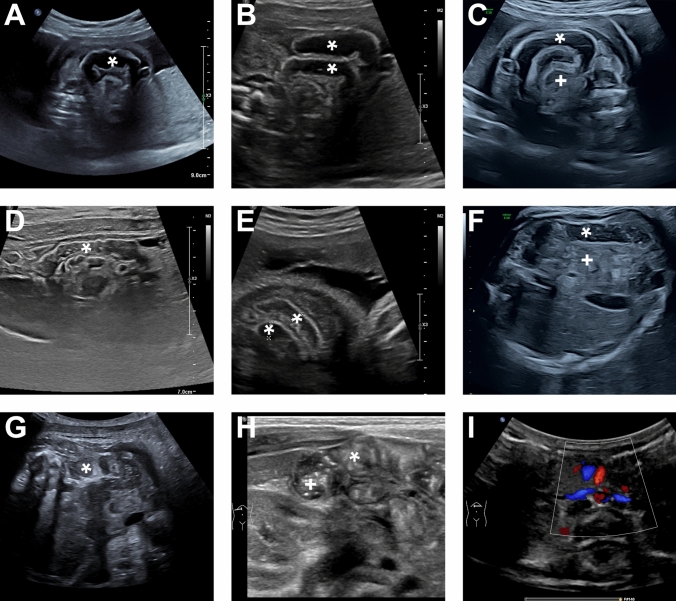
Fetal volvulus with typical prenatal ultrasound findings at 25 weeks of gestation without further symptoms. Due to extreme prematurity, conservative management was the rationale. Sonographic follow-up shows spontaneous detorsion of the volvulus and delivery was at term (38.3 weeks of gestation) without further problems. A Fetal ultrasound at a gestational age of 25 weeks. A dilatation (*) of small bowel loops up to 10 mm was noted in the lower abdomen, with a hyperechogenic bowel wall and detectable peristalsis. The bowel loops are arranged in a rounded, coiled manner (*), consistent with the appearance of a whirlpool sign, raising suspicion of a volvulus. B Fetal ultrasound at a gestational age of 25 weeks showing coffee bean sign (*) suspecting fetal volvulus. C Fetal ultrasound at a gestational age of 25 weeks showing coffee bean/whirlpool sign (* and +) suspecting fetal volvulus. D Fetal ultrasound at a gestational age of 28.6 weeks: The previously observed imaging findings suggestive of a volvulus have markedly regressed. Bowel dilatation was less pronounced (6 mm*), hyperechogenic bowel areas were only focally visible, and there was no evidence of free intra-abdominal fluid. E Fetal ultrasound at a gestational age of 31.6 weeks showing regression of bowel dilatation with normal bowel diameter. A short segment still showed areas with mildly increased wall echogenicity and discreet luminal dilatation. No signs of a clinically significant obstruction were observed. F and G Fetal ultrasound at a gestational age of 36.6 weeks showing normal bowel anatomy with normal bowel diameter (*) and normal mesentery (+). The bowel loops showed mildly increased peristaltic activity. H Postnatal ultrasound showing normal bowel anatomy with normal bowel diameter (*) and bowel filled with stool (+). I Postnatal ultrasound showing normal anatomy of the superior mesenteric vein and artery without sign of torsion
